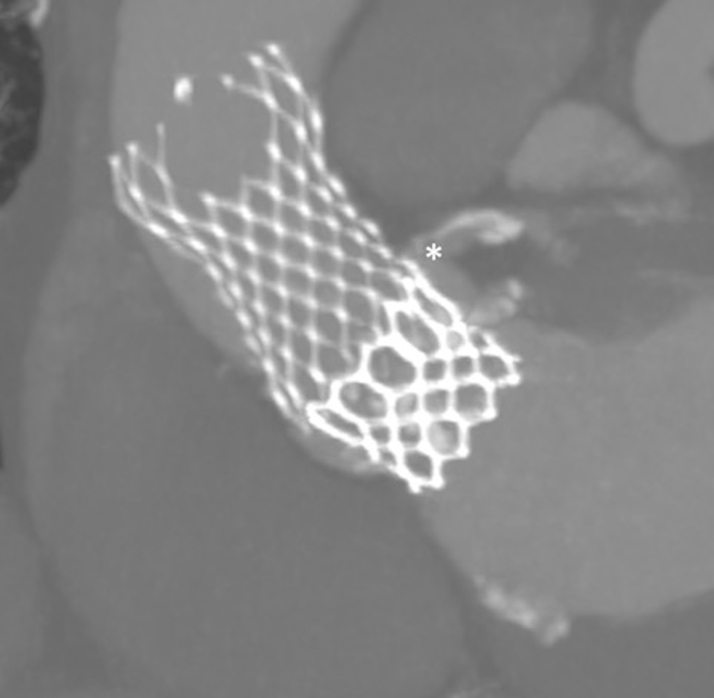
CT Scan, Maximum Intensity Projection The image shows the overlap between the Sapien valve and the Evolut skirt, in front of the left main artery (∗). CT = computed tomography.
